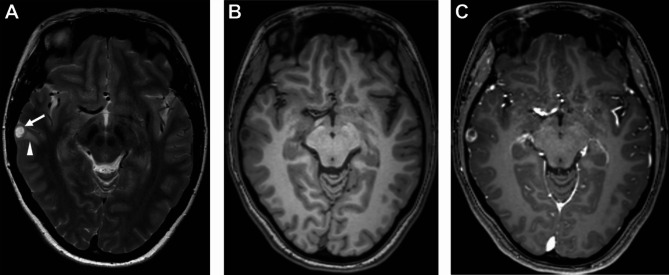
T2 weighted MRI shows a well-defined partially cystic lesion in the right middle temporal gyrus (arrow) with small peritumoral edema (arrowhead) (A). Native (B) and contrast enhanced (C) T1 weighted MRI show a circular contrast enhancement of the lesion
